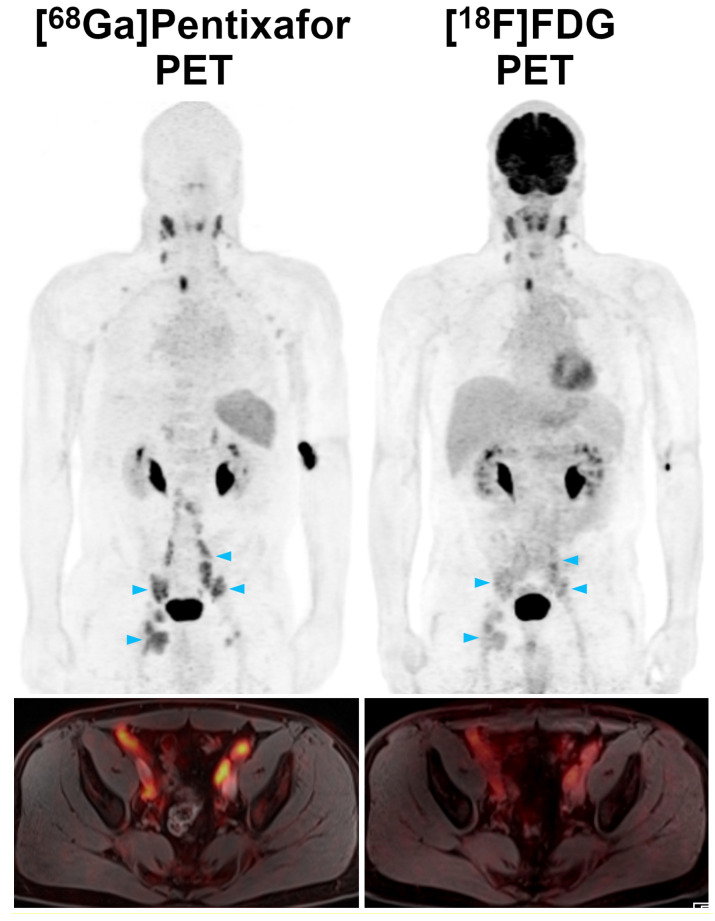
Pre-therapeutic [^68^Ga]Pentixafor-PET/MRI and [^18^F]FDG-PET/MRI of a 52-year-old male MCL patient. Several infradiaphragmatic nodal lymphoma manifestations (blue arrowheads) that show strong [^68^Ga]Pentixafor uptake on PET (top) and fused color-coded PET/MRI (bottom) show only moderate-to-low [^18^F]FDG uptake that does not, or only slightly, exceed the [^18^F]FDG uptake of the liver. Consequently, measured tumor-to-background ratios (TBR_blood_, TBR_liver_) are higher for [^68^Ga]Pentixafor-PET than for [^18^F]FDG-PET.
